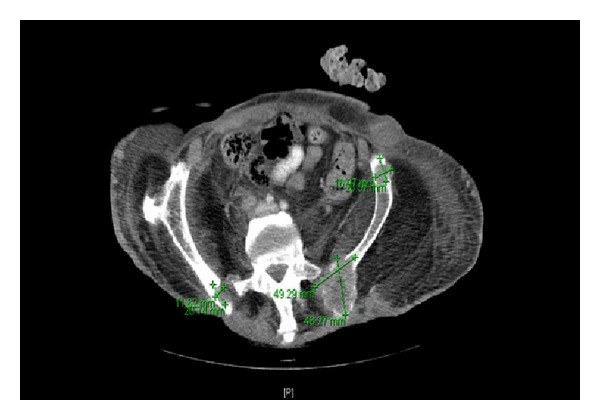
Computed tomography (CT) scan of the abdomen and pelvis demonstrated soft tissue masses with bony destruction of the iliac spines (green lines demarcate bony metastases).
